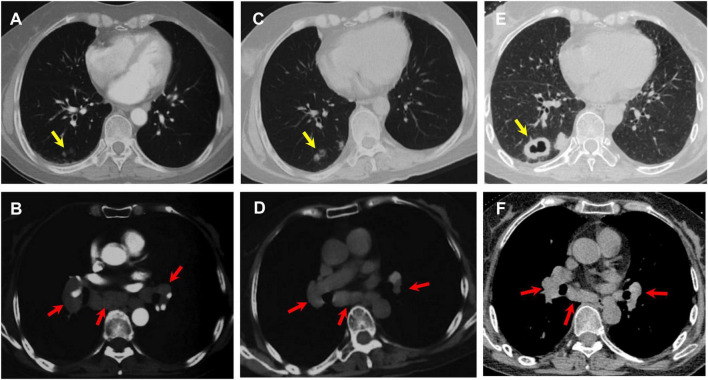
(A,B) Chest CT scan at the onset of disease showing mediastinal and hilar lymphadenopathy (red arrows), along with small nodules in the right lower lobe (yellow arrows). (C,D) Chest CT scan during glucocorticoid treatment demonstrating the lymph nodes decreased in size (red arrows), while nodules in the right lower lobe increased in size (yellow arrows). (E,F) Pre-admission outpatient chest CT scan revealing the nodular shadows progressed in size with cavity formation (yellow arrows).
